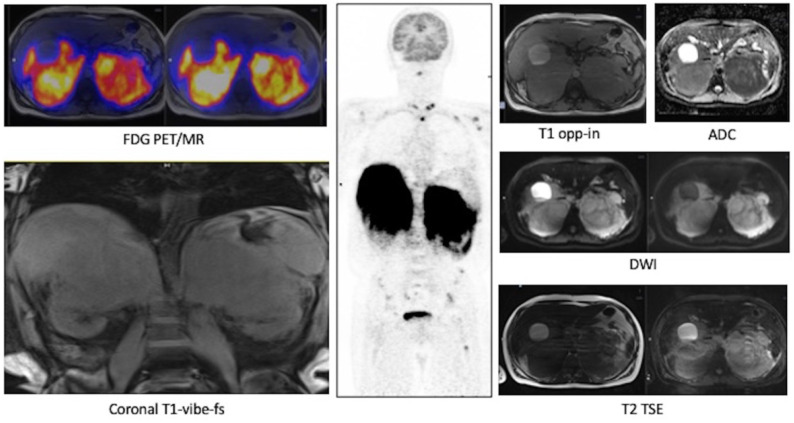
Case #1. FDG PET/MR images.
